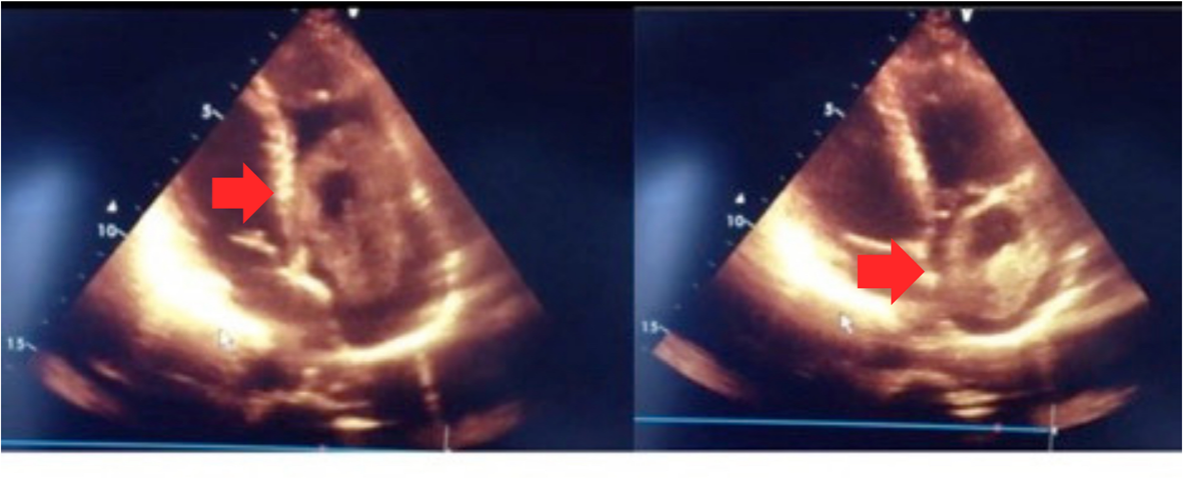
Transthoracic echocardiogram. Longitudinal view of the transthoracic echocardiogram showing a diastolic prolapse into the right ventricle (red arrows)
